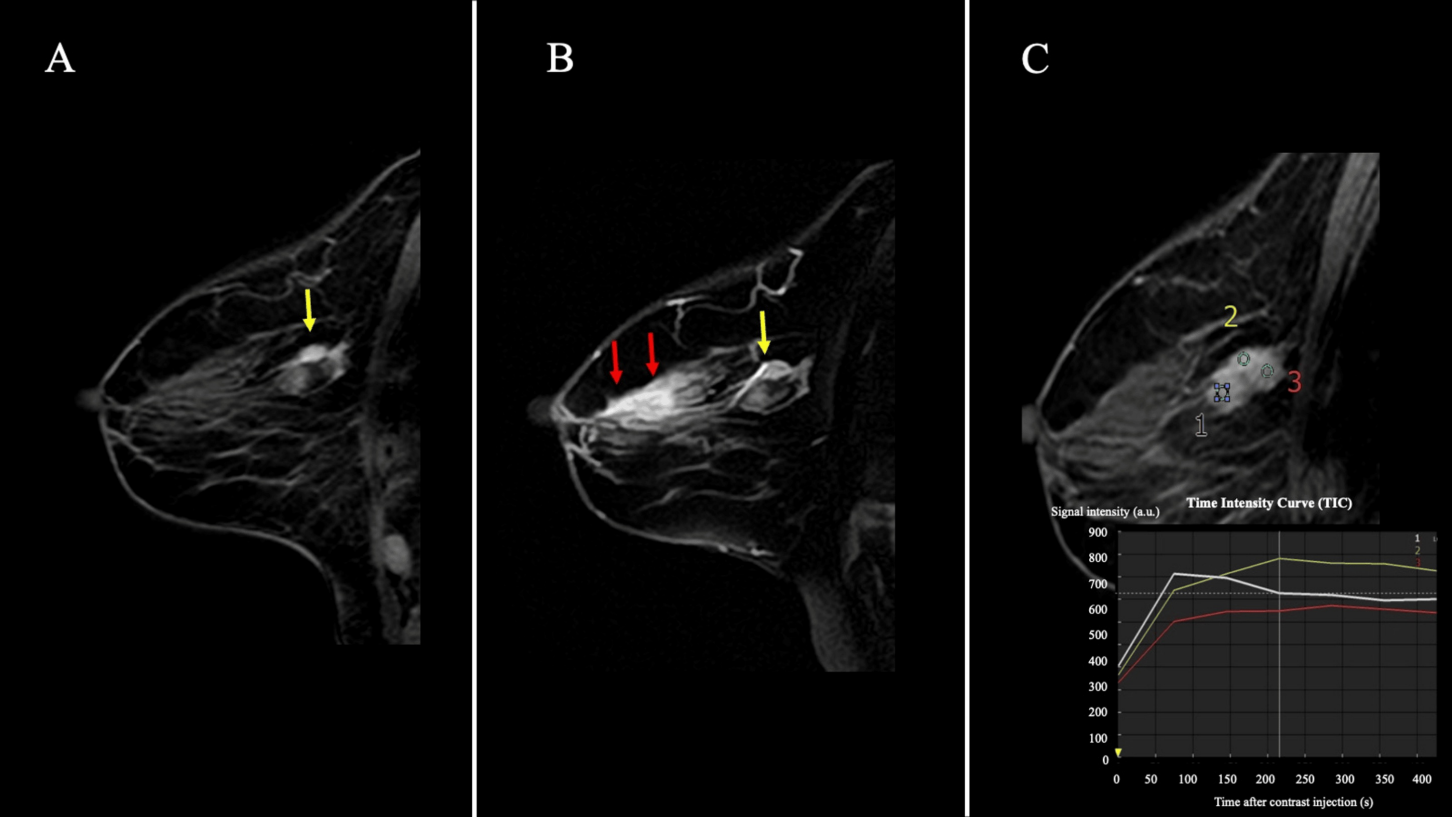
Magnetic resonance imaging (MRI) findings (A) Dynamic early phase on contrast-enhanced fat-suppressed T1-weighted imaging (T1WI). The main lesion is indicated by a yellow arrow. (B) Delayed phase image showing the main tumor (yellow arrow) and intraductal spread toward the nipple (red arrow). (C) Regions of interest (ROIs 1-3) and the corresponding time-intensity curves (TIC). X-axis: Time after contrast injection (s). Y-axis: Signal intensity (arbitrary units, a.u.).
